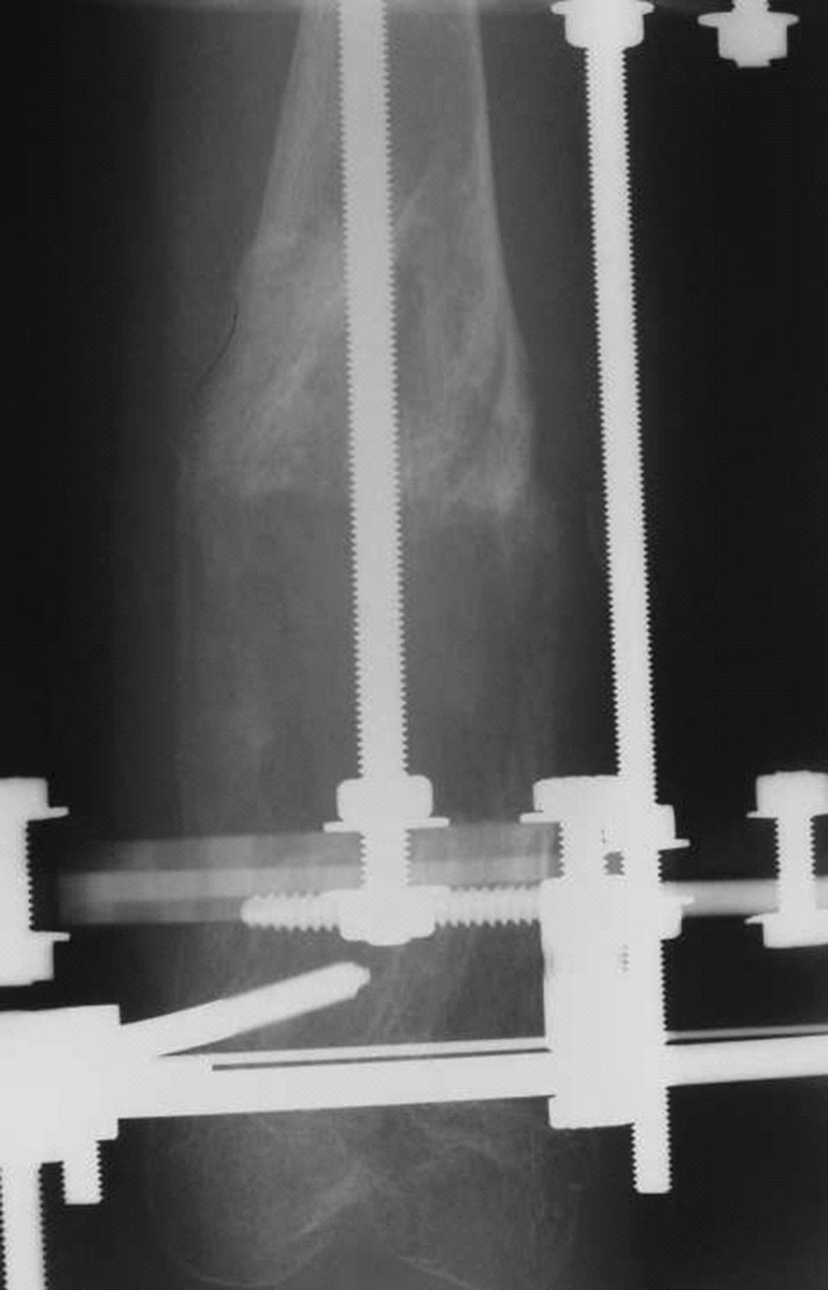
Radiograph of deformity correction of tibia using external fixation
